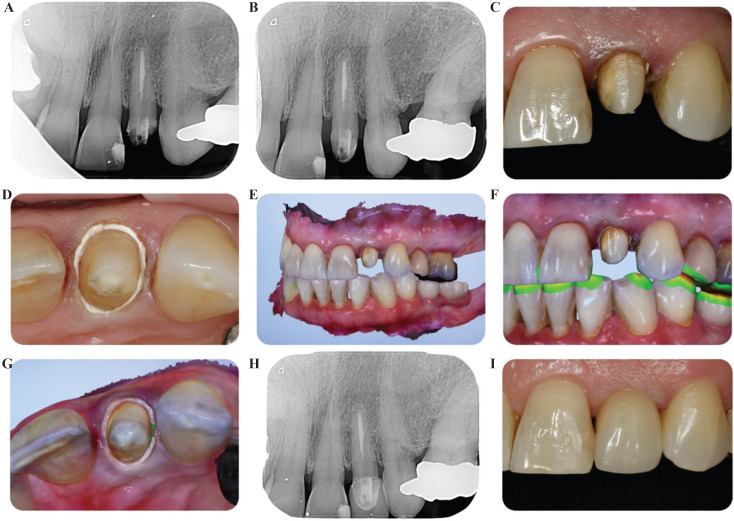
(A) Preoperative periapical radiograph. (B-C) Periapical radiograph and photograph of the preparation. (D) PTFE tape placed into the sulcus. (E-F) STL image of the scan. (G) STL image of the prepared tooth with the PTFE tape into the sulcus. (H-I) Periapical radiograph and final photograph with the bonded crown.
